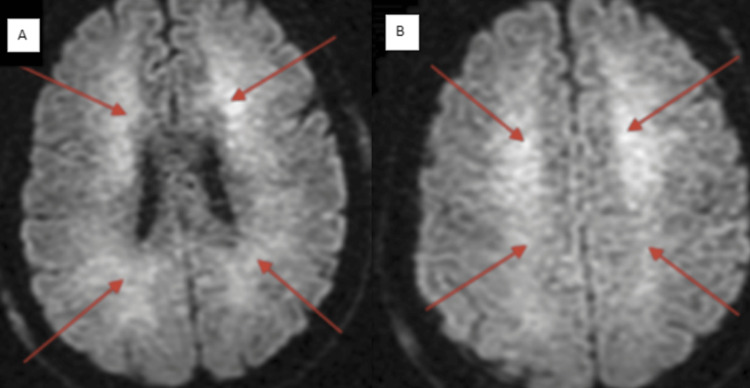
Brain MRI showing a diffuse abnormal DWI signal in a subcortical manner (A) and (B) in a patient with a positive GAD antibody (red arrows). DWI: diffusion-weighted imaging; GAD: glutamic acid decarboxylase.
